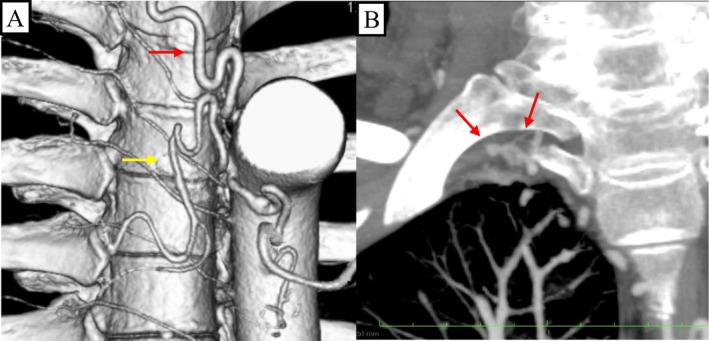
(A) Three‐dimensional CTA demonstrated mild dilation of the right bronchial artery (2.1 mm at the ostium) (yellow arrow) and marked dilation and tortuosity of the right second intercostal artery (2.5 mm) (red arrow). (B) Coronal images from CTA showed marked dilation and tortuosity of the right supreme intercostal artery (2.7 mm) (red arrow).
